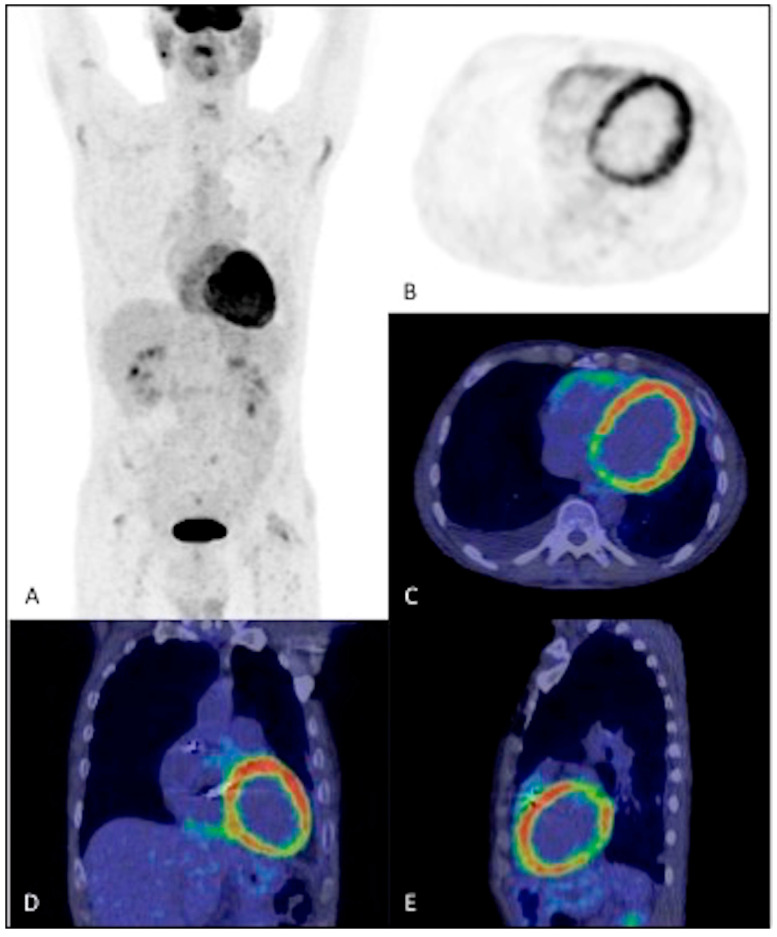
^18^F-FDG PET/CT of a 66-year-old man with a dual-chamber ICD with suspected CIEDI. (A) MIP, (B) axial PET, (C) axial fusion, (D) coronal fusion, and (E) sagittal fusion images demonstrate widespread glucose uptake throughout the myocardium, indicative of poor adherence to the dietary protocol for myocardial suppression; these images are considered of poor quality. The ^18^F-FDG PET/CT was considered negative for CIEDI but it was revealed to be a false negative.
